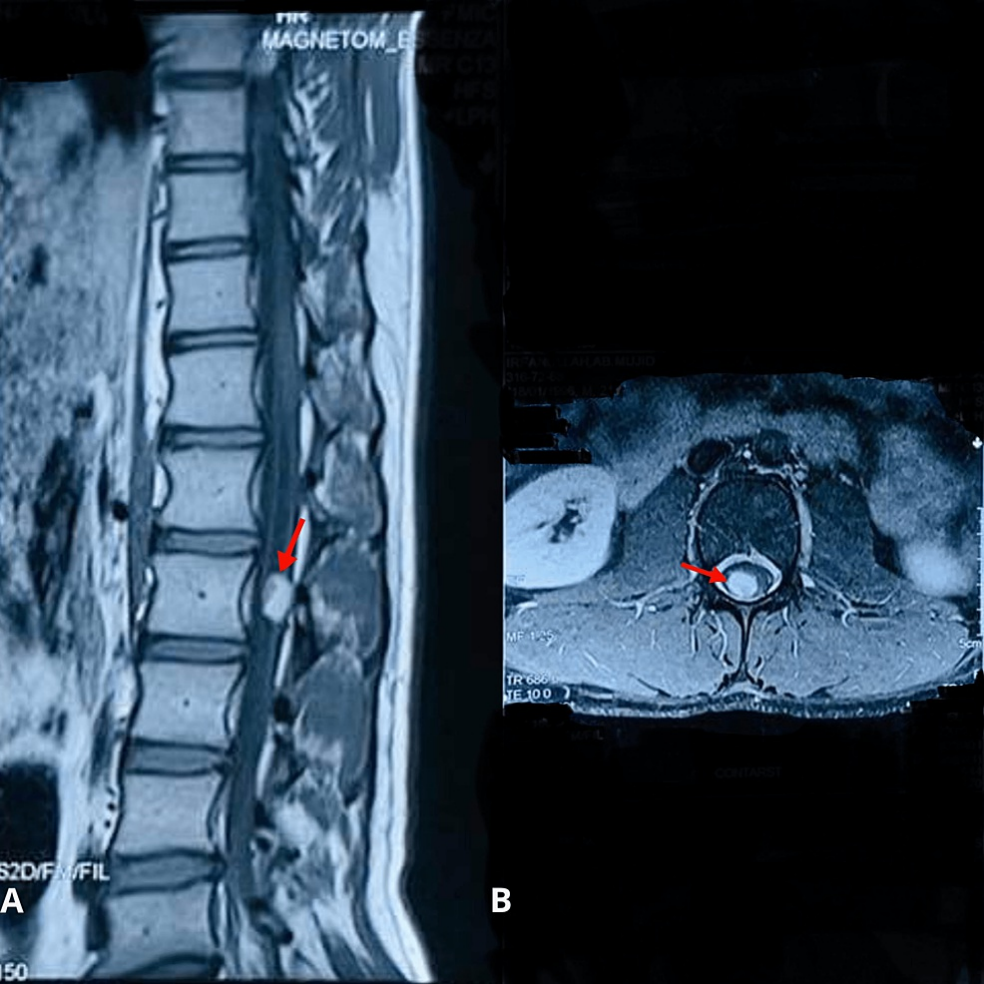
MRI of the spine with IV contrast showing a sagittal view (A) and axial view (B) of the lesion at level L2 (red arrows). IV: Intravenous
